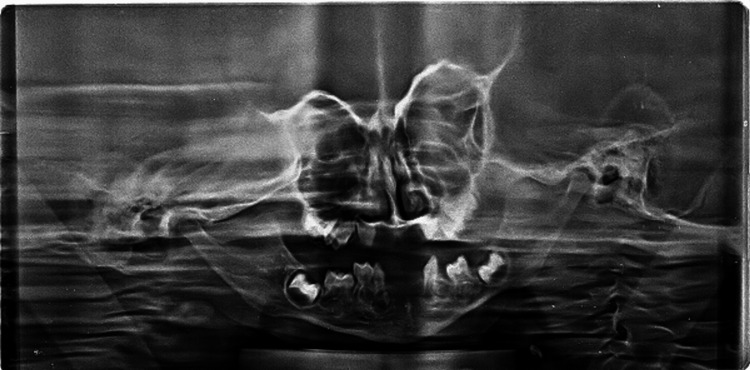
Orthopantomogram (OPG) view showing the presence of tooth buds 83, 84, 85, 46, 73, 75, 36, 16, 53, 55, 63, 65, and 26 and the absence of other tooth buds
